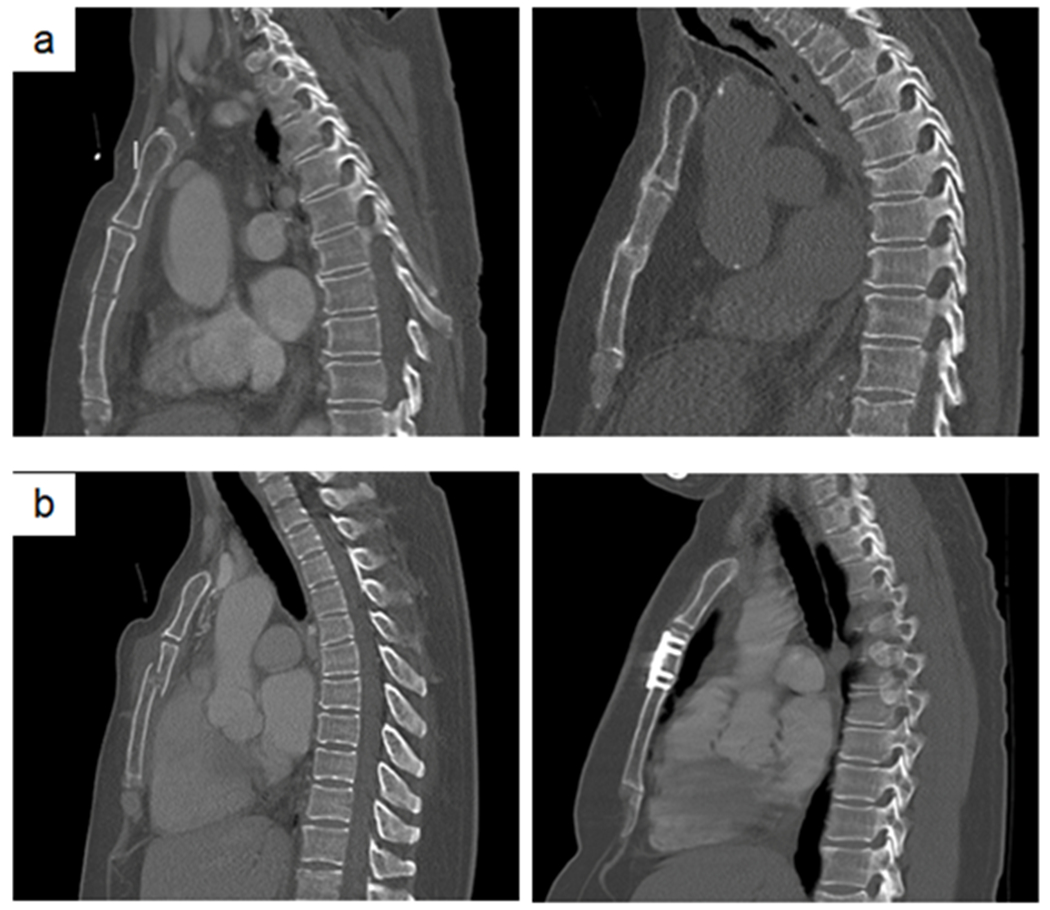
Chest CT images of traumatic sternal fractures. a- Conservatively treated sternal fracture in a 74-year old female patient, at trauma (left) and at follow-up after seven years (right). b- Surgically treated sternal fracture in a 50-year old male patient, at trauma (left) and at follow-up after two years (right).
